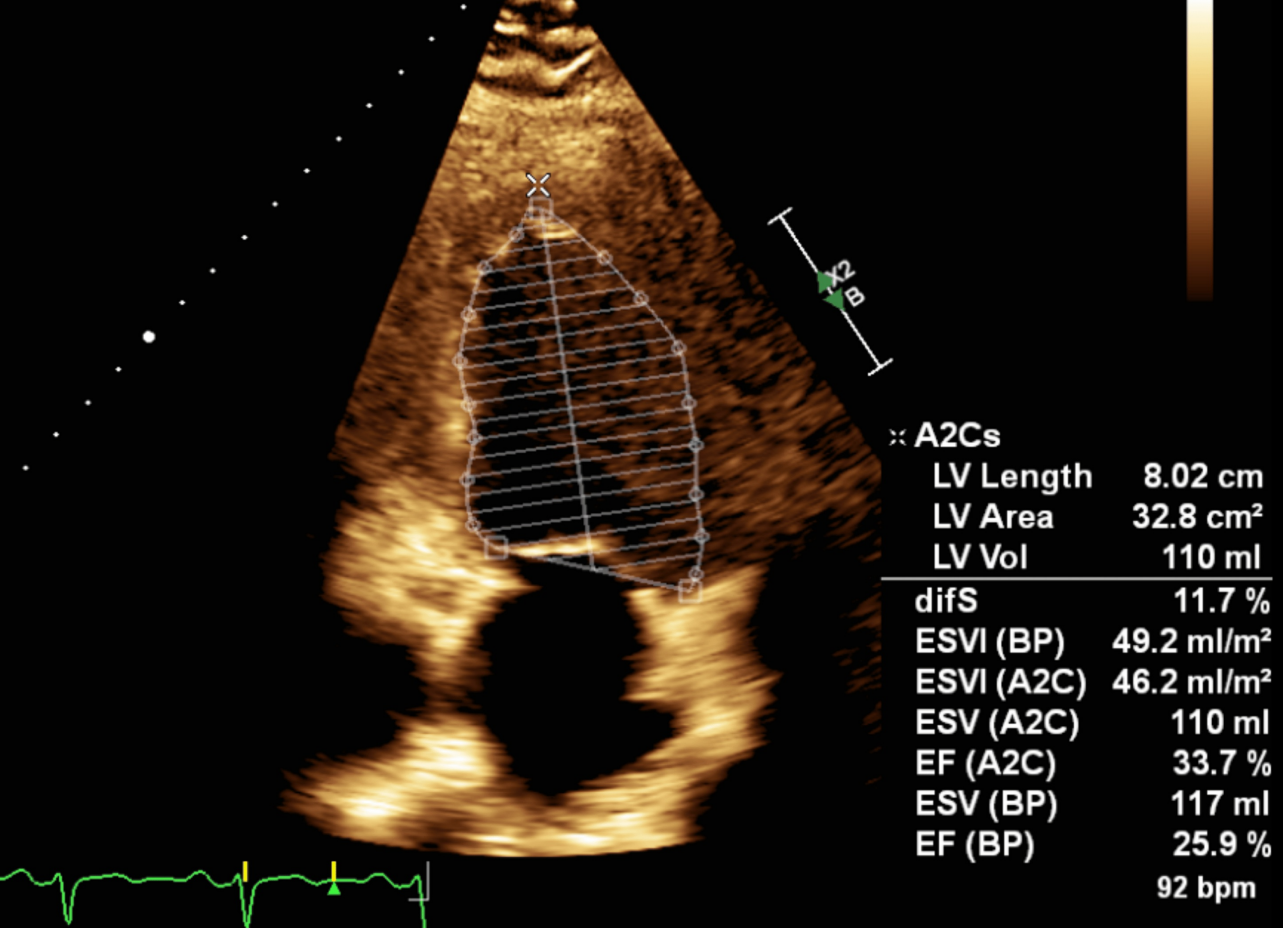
Transthoracic echocardiography (TTE) Apical two-chamber (A2C) view showing left ventricular (LV) volume assessment using Simpson's biplane (BP) method of discs. Measurements demonstrate reduced left ventricular ejection fraction (EF) of 30-35% with increased end-systolic volume (ESV) and end-systolic volume index (ESVI), consistent with impaired LV systolic function following acute myocardial infarction.
